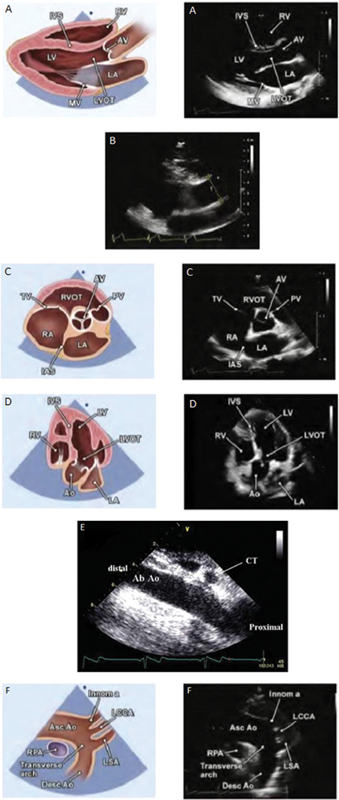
Canonical views on transthoracic echo (TTE): (
